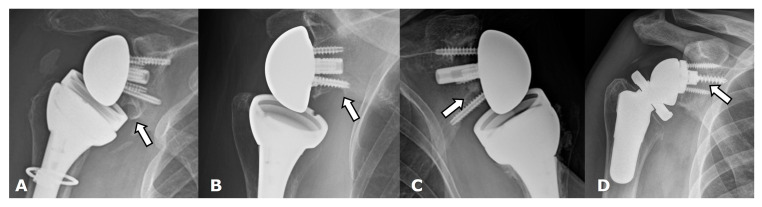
Frontal radiographs. Scapular notching grading by using Sirveaux Classification in four different patients with a reverse total shoulder arthroplasty. (A). Grade 1. (B). Grade 2. (C). Grade 3. (D). Grade 4.
